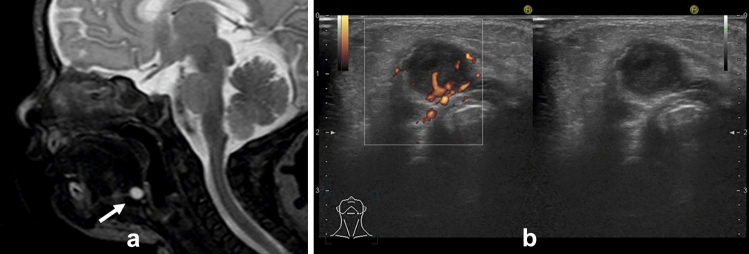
a One-year-old boy with the thyroglossal duct cyst. T2 weighted image incidentally revealed cyst which showed high signal intensity in front of the hyoid bone. b Two-year-old boy with the thyroglossal duct cyst. Ultrasonography revealed hypoechoic cyst in front of the hyoid bone. Echogenicity was slightly higher than simple cyst and flow was detected on Doppler ultrasonography due to infection of the thyroglossal duct cyst
